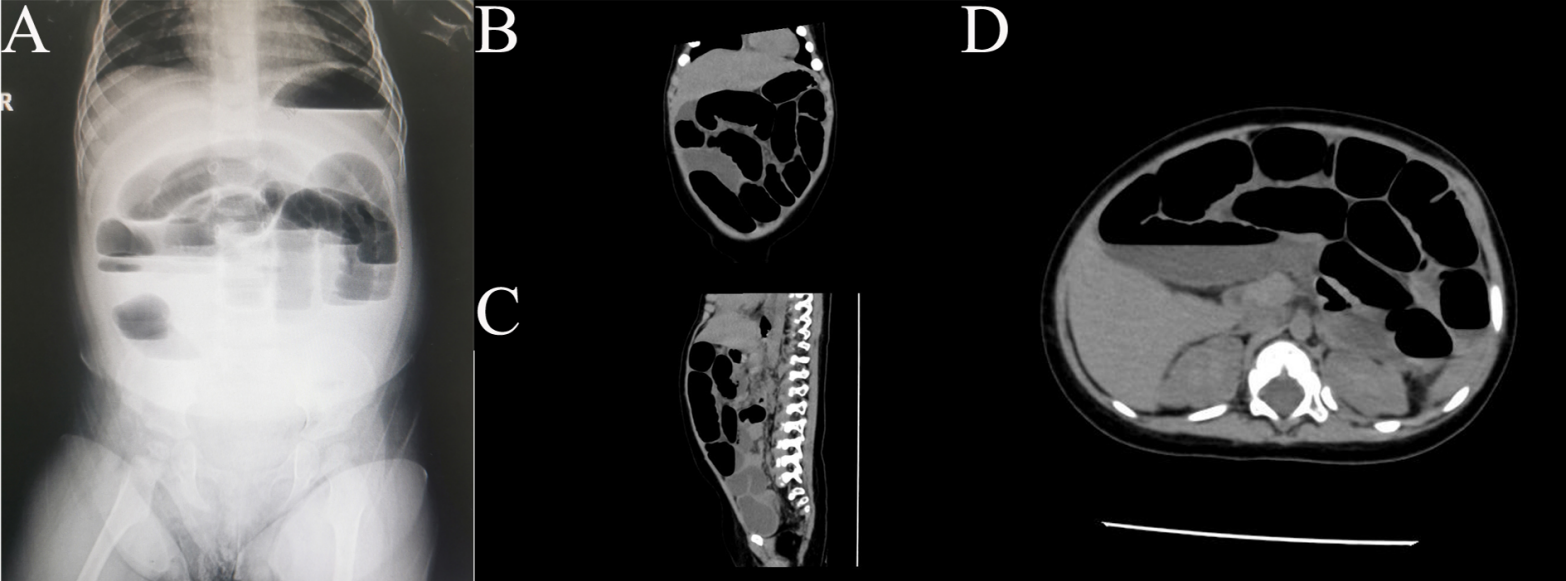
Imaging findings of small bowel obstruction Due to Shine-Muscat grape in an infant. (A) An upright abdominal radiograph of the infant showing signs of small bowel obstruction, characterized by multiple dilated loops of bowel and air-fluid levels suggestive of a mechanical obstruction. (B–D) Abdominal CT scans in various planes (sagittal, coronal, and axial) further confirm the presence of small bowel obstruction. The images demonstrate dilated intestinal loops with a transition point, suggesting a luminal obstruction caused by the ingestion of a Shine-Muscat grape. No evidence of perforation or additional masses is observed.
